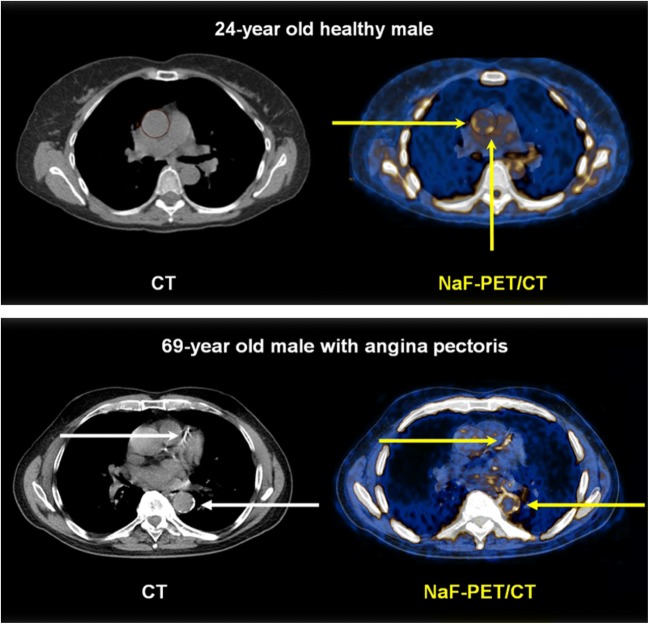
Abnormal arterial wall NaF uptake has been observed down to the age of 20 and is most often present in the absence of CT-calcification. Upper panel: NaF uptake (right) in the thoracic aorta (yellow arrows) of a 24-year-old symptom-free male with no CT-visible calcification (left). Lower panel: 69-year-old male with angina pectoris and CT-visible calcification in the left anterior descending coronary artery and the descending thoracic aorta (white arrows, left) and NaF uptake in the same arteries, incongruent with CT-calcifications and with far greater circumferential extension in the aorta (yellow arrows, right) (images from material of references 23 and 30)
